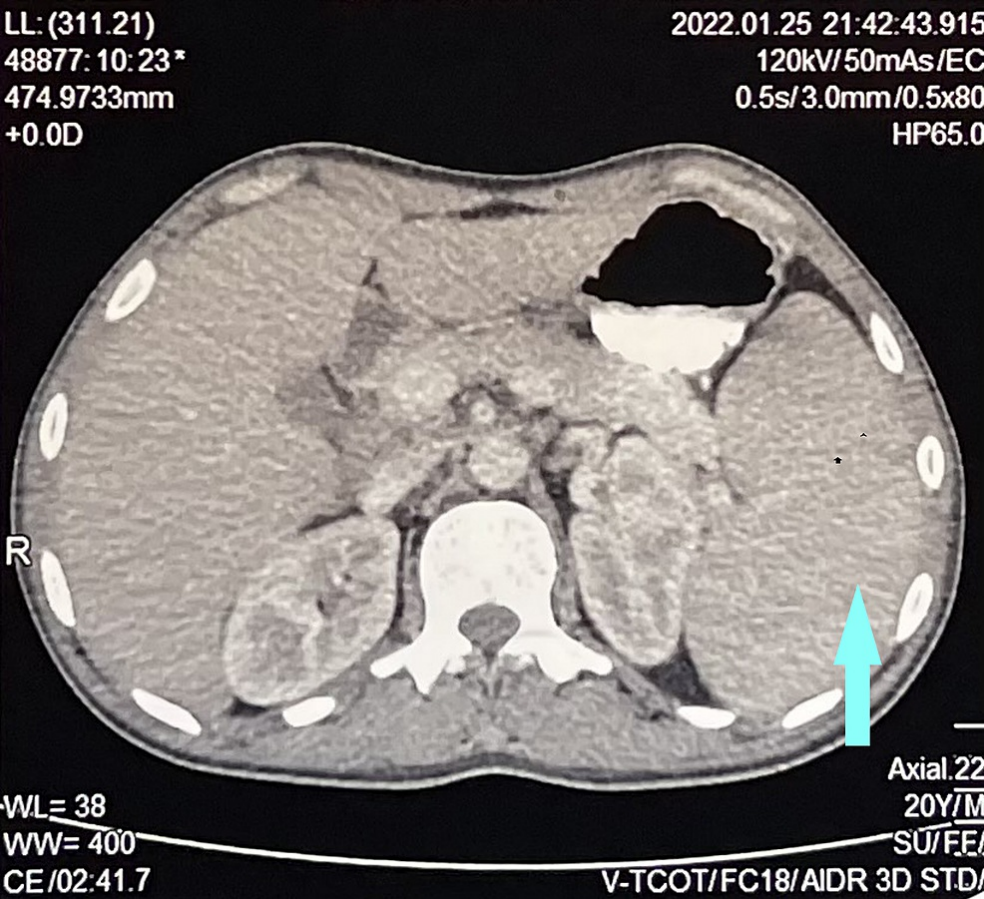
CT scan image of the patient's abdomen showing enlarged spleen
